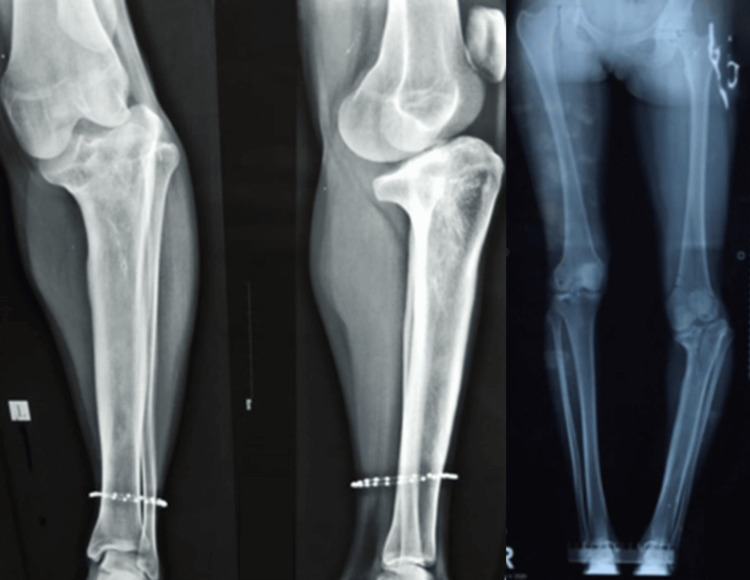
Preoperative X-rays of the aforementioned deformity.
